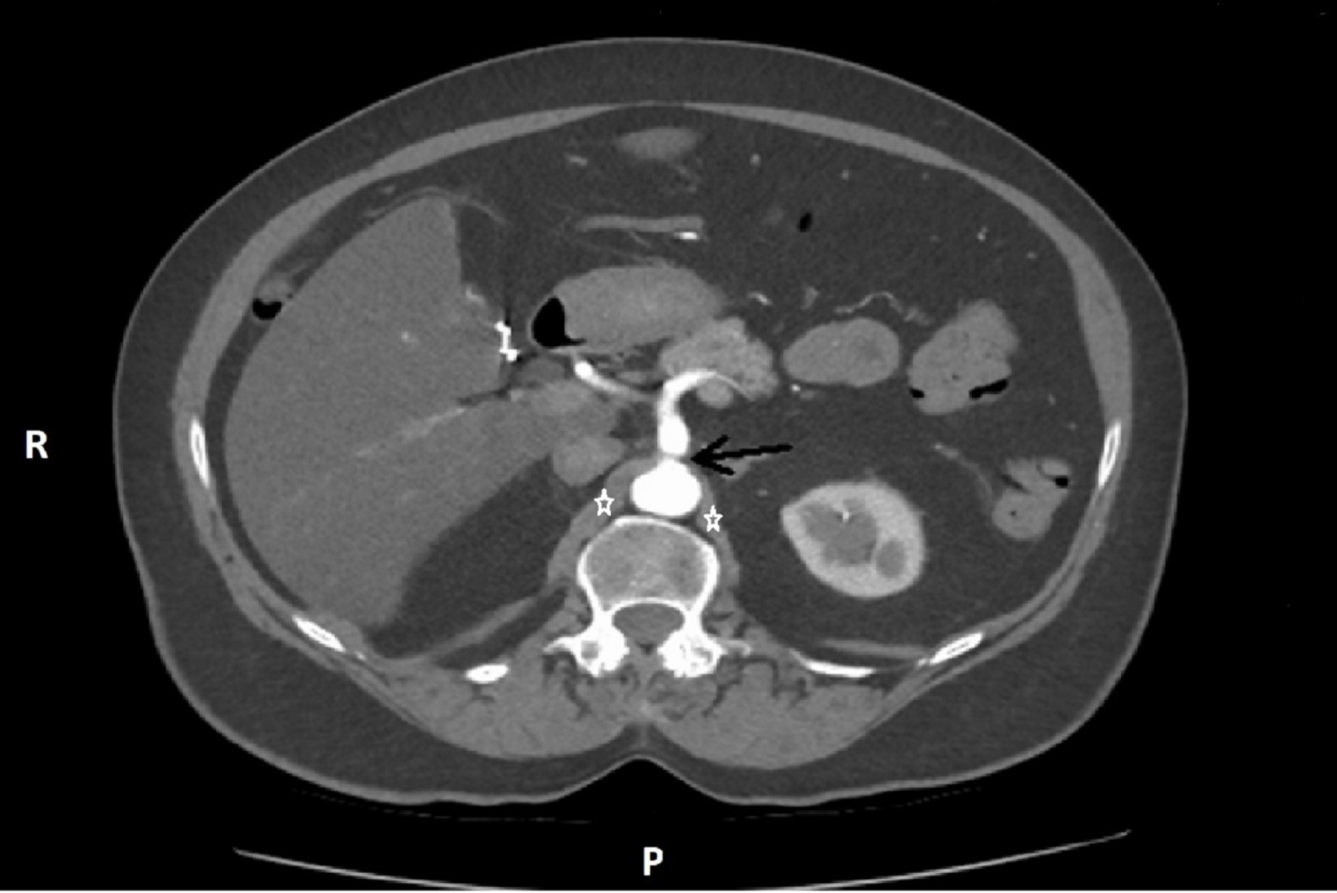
Axial CT angiogram of the abdomen. The image at the level of origin of celiac trunk (black arrow), in which a linear hypodense structure (star) separates it from the abdominal aorta and indicates the arcuate ligament compression
